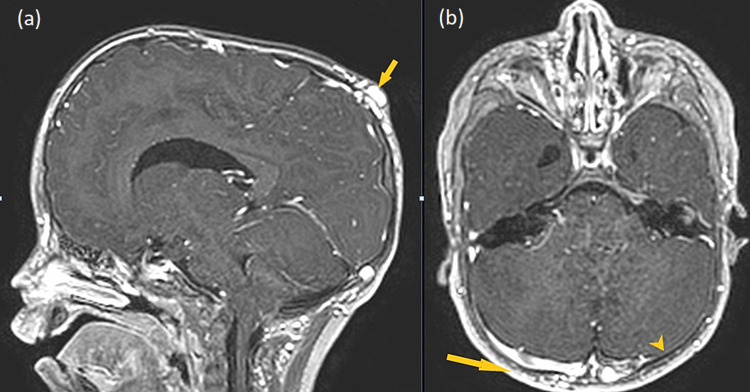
Sagittal (a) and axial (b) T1W post-contrast images. The images are showing prominent transcranial emissary veins traversing cranial defects in parietal and occipital regions, communicating with underlying superior sagittal sinus and torcular Herophili to supply scalp venous mass (yellow arrows). Hypoplastic left transverse sinus (yellow arrowhead). T1W: T1-weighted
